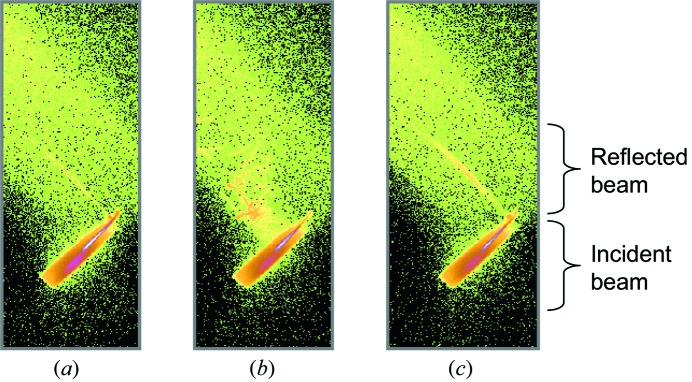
Detector images of the X-ray beam reflected by the phosphate buffer solution: (*a*) before protein injection, (*b*) 2 s and (*c*) 12 s after protein injection.
